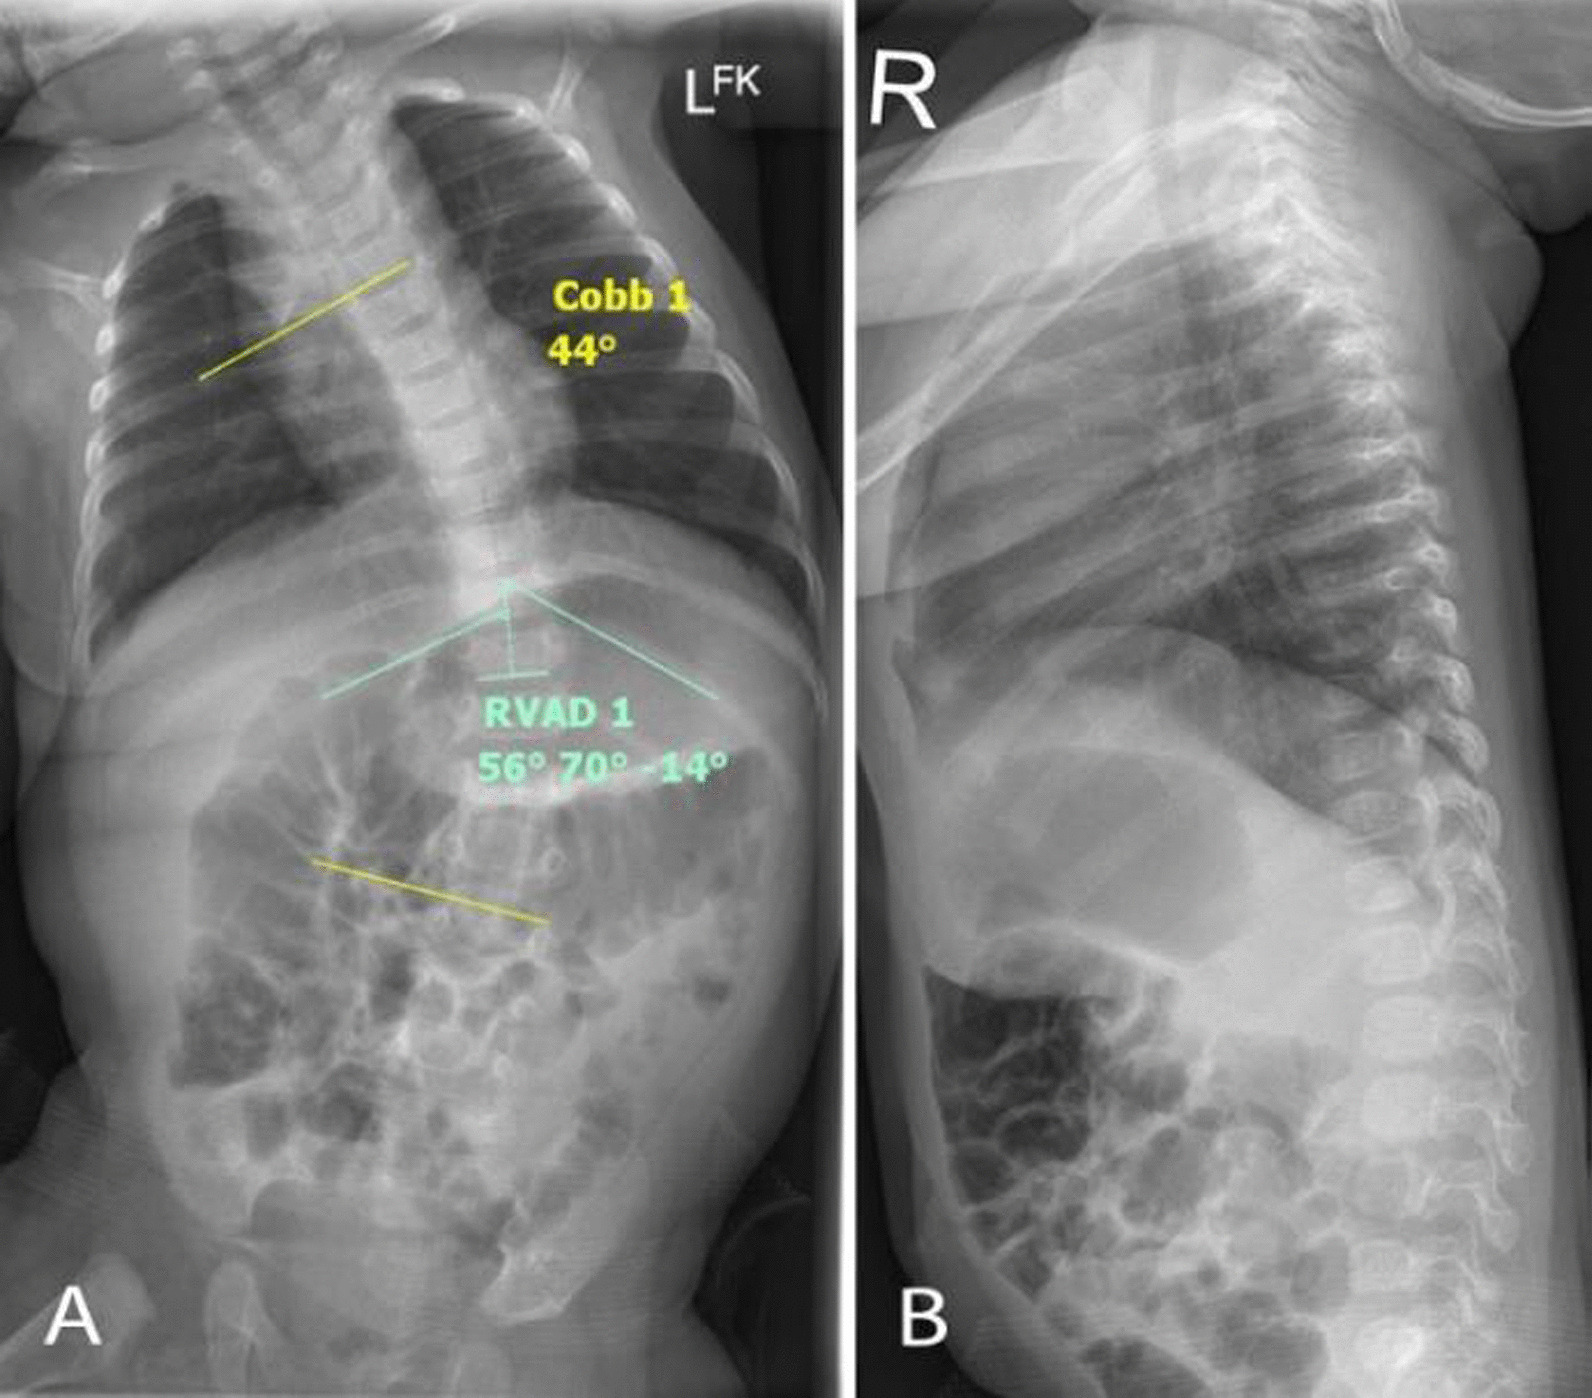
Patient x-ray findings with Cobb angle and RVAD. A Supine anteroposterior. B Right lateral decubitus
